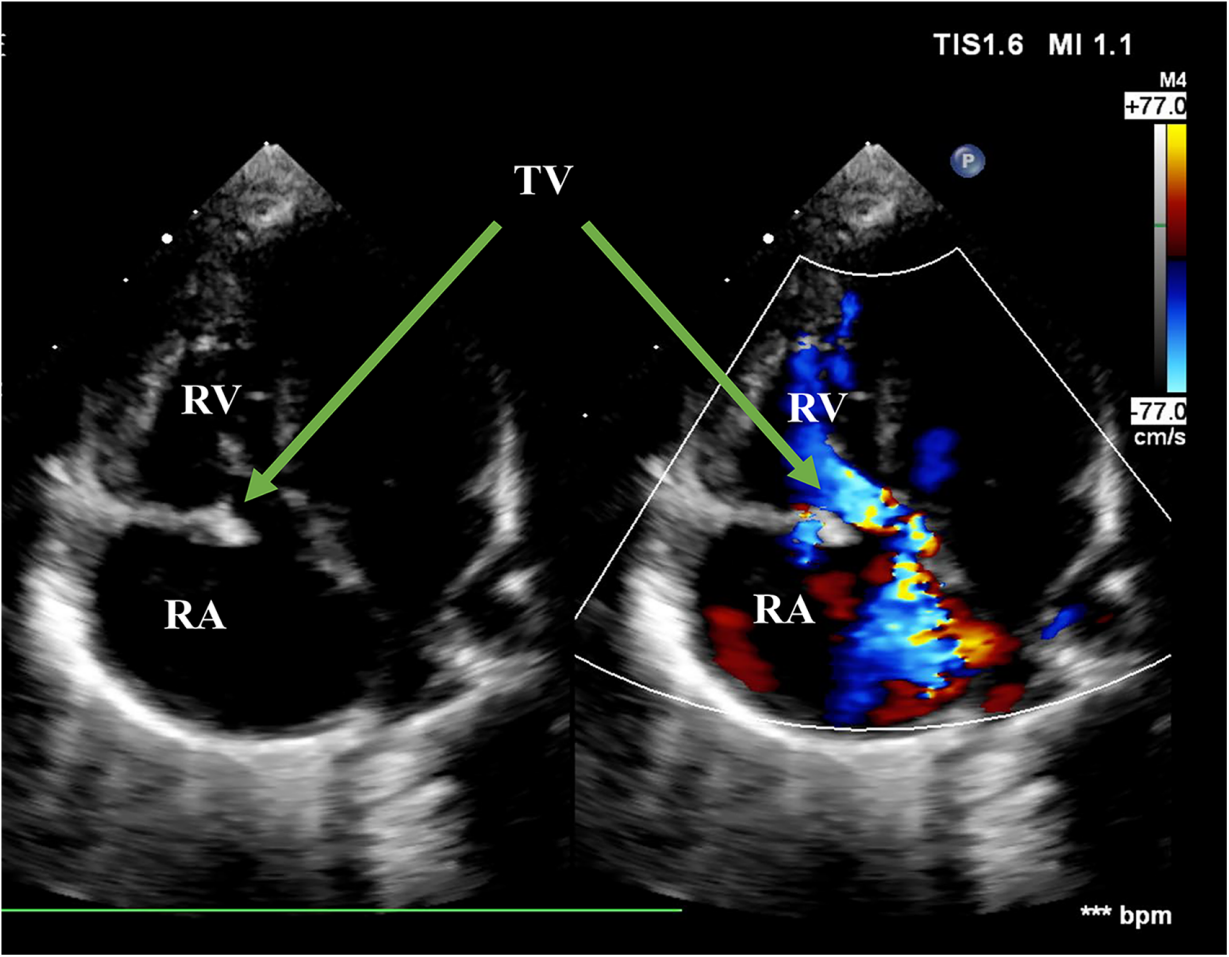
Echo before surgery in the A4C view. The picture showed flailed anterior leaflet prolapsing into the right atrium with a hyperechogenic mass. The green arrows pointed at flailed anterior leaflet of the tricuspid valve. RA, right atrium; RV, right ventricle; TV, tricuspid valve; A4C, apical four-chamber.
